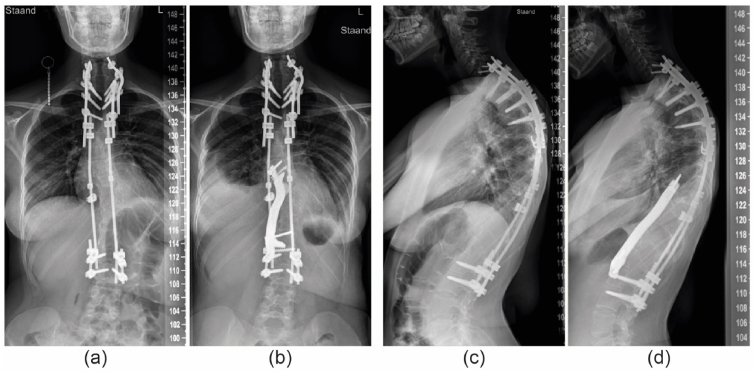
Patient 4; (a) Preoperative radiograph showing the posterior instrumentation and dystrophic thoracolumbar area. (b) after insertion of the anterior titanium strut prosthesis. (c) and. (d) lateral radiographs at the same timepoints.
